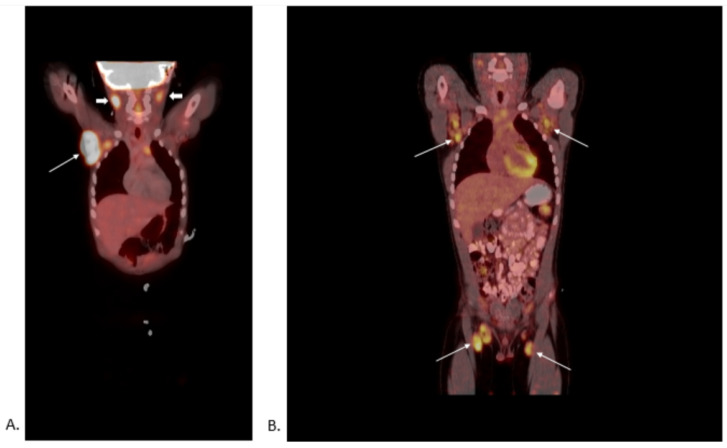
(A). PET CT skull base to midthigh showing multifocal lymphadenopathy with the largest, most metabolically active node in the right axilla (arrow) Deauville score of 4. Other areas of increased uptake were noted in cervical lymph nodes (bold arrow), thymus, peri-aortic, retroperitoneal, and inguinal nodes, Deauville score of 4; 2 times the liver SUVmax. (B). PET CT of skull base to midthigh showing persistent diffuse lymphadenopathy performed two years after initial presentation. Compared to an earlier study two years back, there was a stable to a mild decrease in metabolic activity in cervical, axillary (arrows) (arrows), iliac, and inguinal (arrows) lymphadenopathy, Deauville score of 4; 2 times the liver SUVmax. There was also stable to mildly increased left iliac lymphadenopathy. All lymph nodes were scored using Deauville criteria (5-point scale-Deauville Score [DS]) with a positive PET defined by tumor residual uptake moderately higher than liver (Deauville score of 4) or 2–3 times the liver SUVmax (Deauville score of 5). SUV-Standardized Uptake Value.
